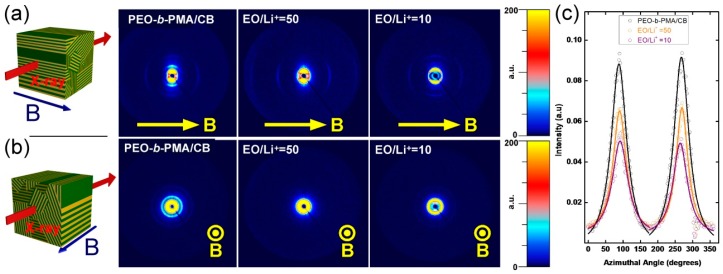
(a) Room temperature 2D SAXS data of EO-MA/CB samples at different EO:Li^+^ ratios, where the field was kept horizontal with respect to the X-ray detector’s plane (top panel) and the corresponding azimuthal intensity profiles of the BCP super structure peak (c). (b) Room temperature 2D SAXS data of the same samples when the field was applied normal with respect to the X-ray detector’s plane (bottom panel).
